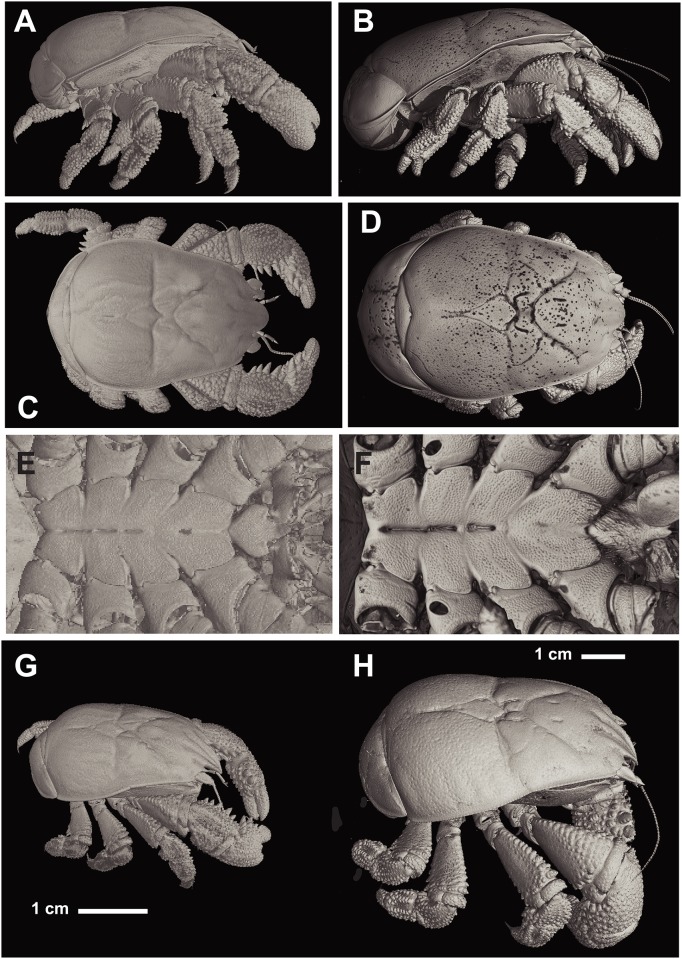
Kiwa tyleri* sp. nov.; paratype, male (NHMUK 2015.2792). A) lateral view; C) dorsal view; E) ventral view, sternal plastron; G) dorso-lateral view; holotype, female (NHMUK 2015.2791) B) lateral view; D) dorsal view, F) ventral view, sternal plastron, with gonopore on first segments of pereopod 3 (arrow); note: sternal plastron with remnants of setae (methodological artefact, see methods section); H) paratype, large male (NHMUK 2015.2793) (all images based on micro-focus X-CT).
